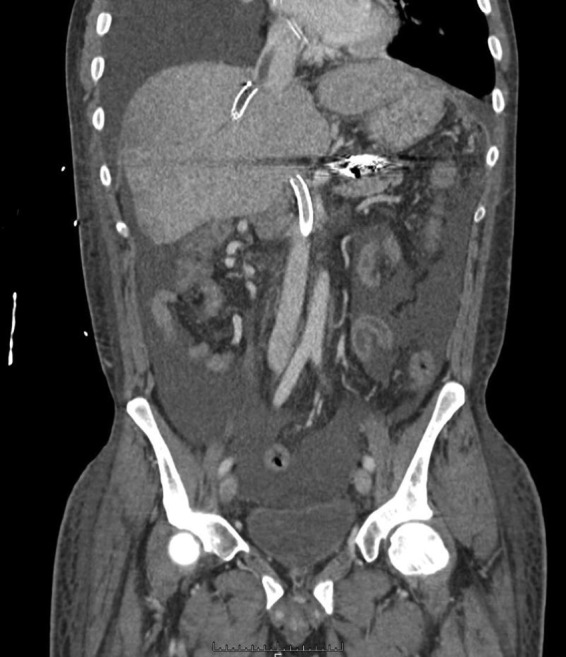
Coronal cut of a CT scan showing the (i) TIPS with the infected thrombus extending to the right atrium, (ii) occluded superior mesenteric vein stent, and (iii) abdominal ascites. TIPS, transjugular intrahepatic portosystemic shunt.
